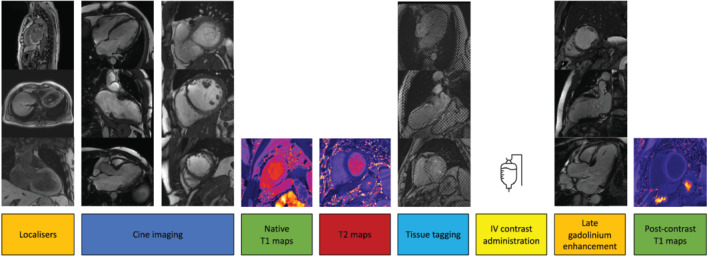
Cardiac magnetic resonance imaging protocol outline. Cardiac magnetic resonance imaging was performed pre‐randomization and at 12 months following double‐blind treatment with sacubitril/valsartan or valsartan. All scans were performed on a single, 3 Tesla Siemens MAGNETOM Prisma scanner. Further details regarding the scan protocol are available in the Supporting Information.
